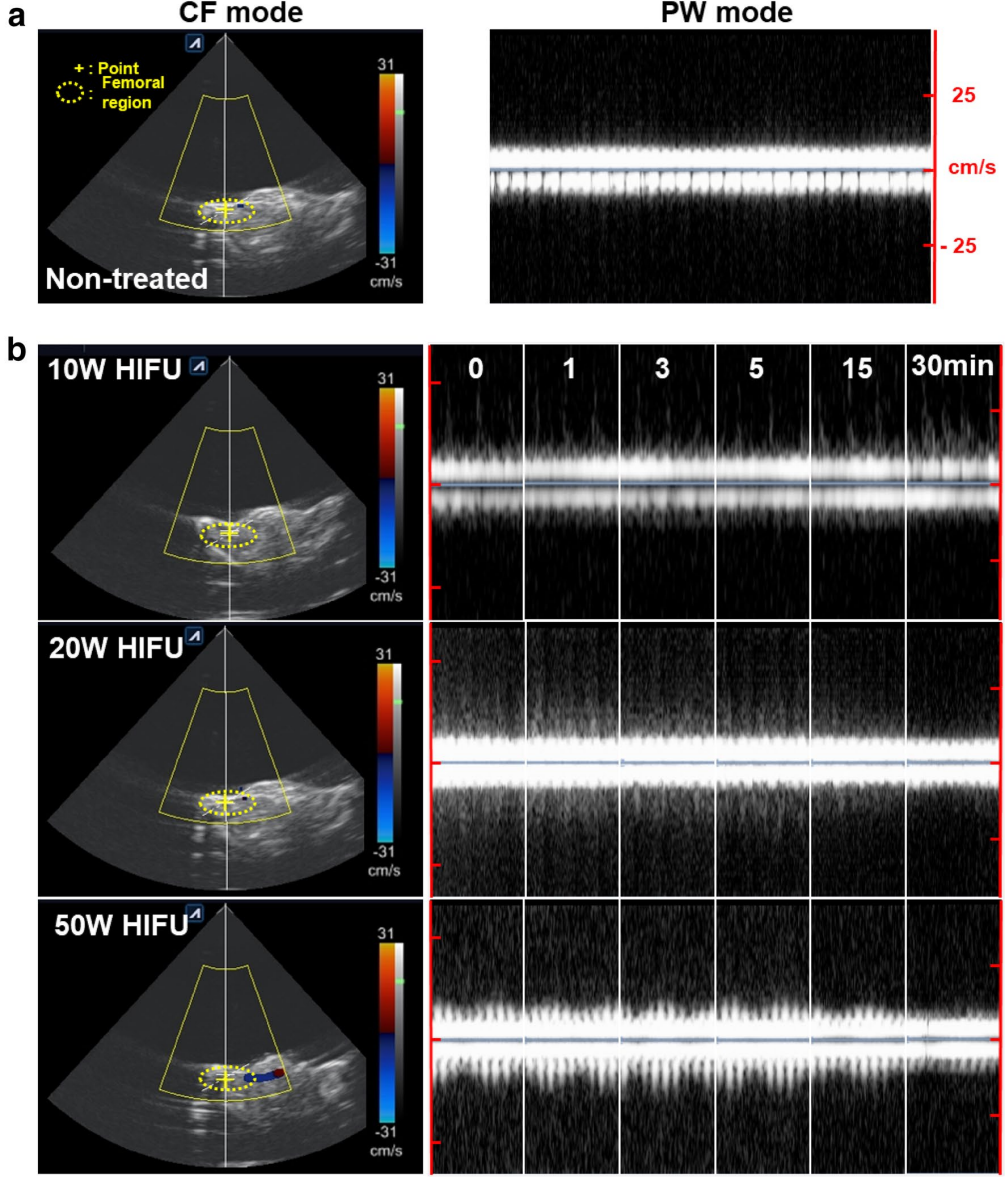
pHIFU-mediated blood flow enhancement. Color-flow Doppler ultrasound image and pulsed-wave Doppler image of a non-treated (0 W pHIFU) and b 10, 20, and 50 W pHIFU-treated femoral tissue. Yellow dot circles indicate the femoral vessel region
